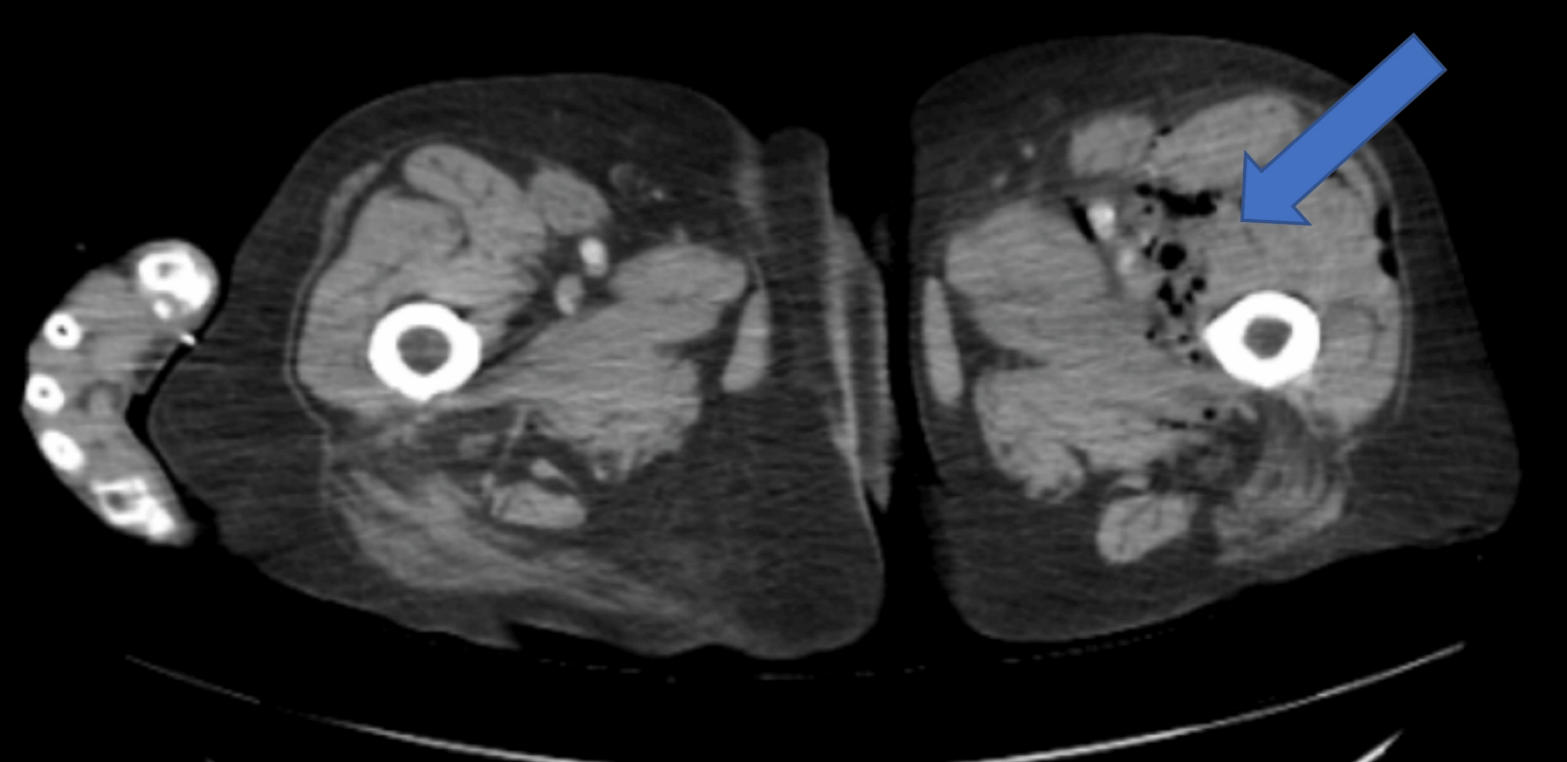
Axial CT demonstrating gas within the thigh musculature, consistent with extension of retroperitoneal infection and necrotizing soft tissue involvement The blue arrow highlights gas within the thigh musculature.
